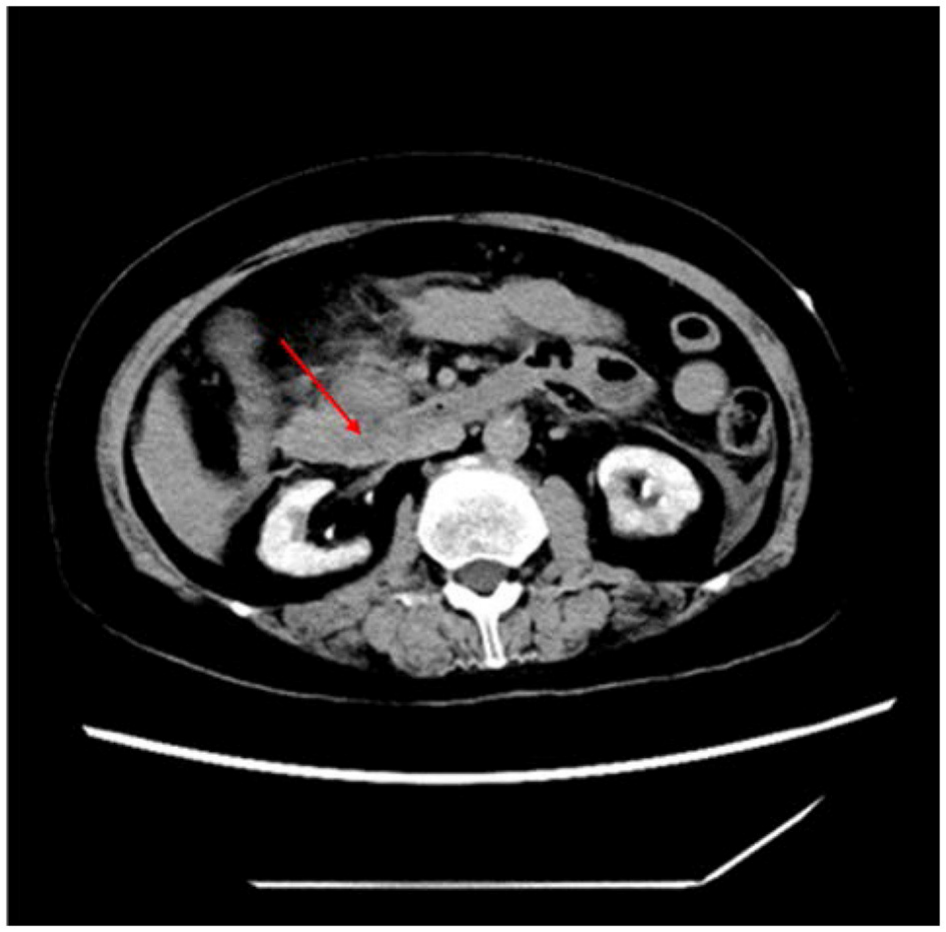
Abdominal CT on admission showed acute pancreatitis with peripancreatic exudation (red arrows).
